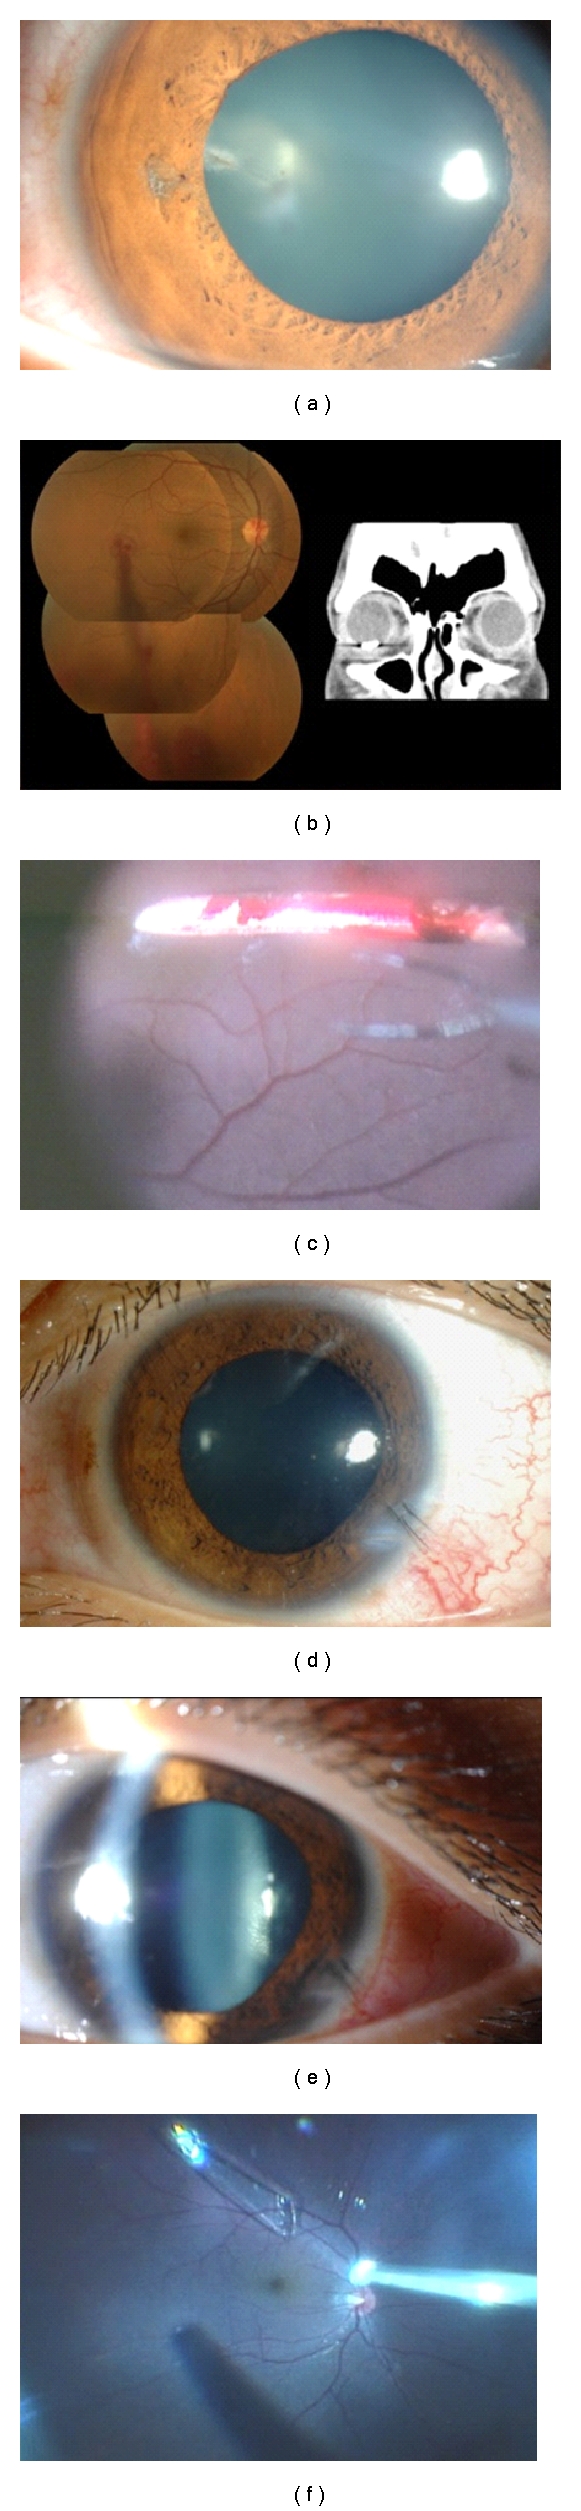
Preoperative slit-lamp photographs, preoperative fundus photograph, preoperative computed tomographic image, and intraoperative photographs of intraocular foreign body (Case 1; (a, b, c), Case 2; (d, e, f)). (a) Preoperative slit-lamp photograph shows a slight penetrating wound in the iris and lens at the 9 o'clock position and a posterior subcapsular cataract at the same position. (b) Fundus photograph showing vitreous haemorrhage and retinal tear with subretinal haemorrhage located on the temporal side of the macula. Computed tomographic image showing a large foreign body. (c) Intraoperative fundus showing a large metallic intraocular foreign body anterior to the retina. (d) External photograph showing the penetrating wound at the 4 o'clock position and the corneal wound was closed by corneal sutures during the initial surgery. (e) Slit-lamp photograph showing that the posterior subcapsular cataract has progressed. (f) Intraoperative fundus photograph showing large glass intraocular foreign body anterior to the retina.
